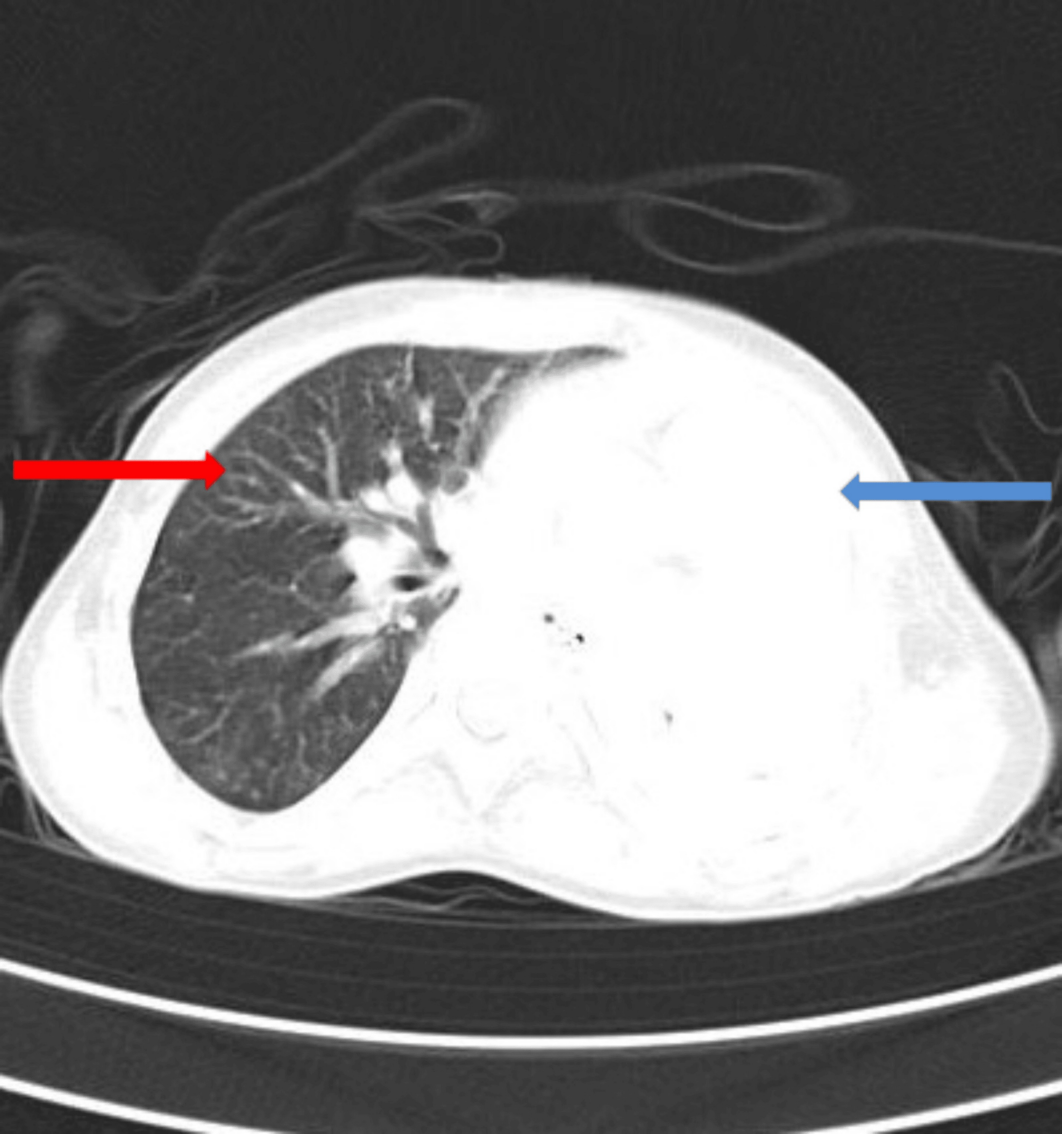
HRCT thorax showing left collapse (indicated by the blue arrow) and right lung consolidation with tree-in-bud appearance (indicated by the red arrow) HRCT: High-resolution computed tomography
